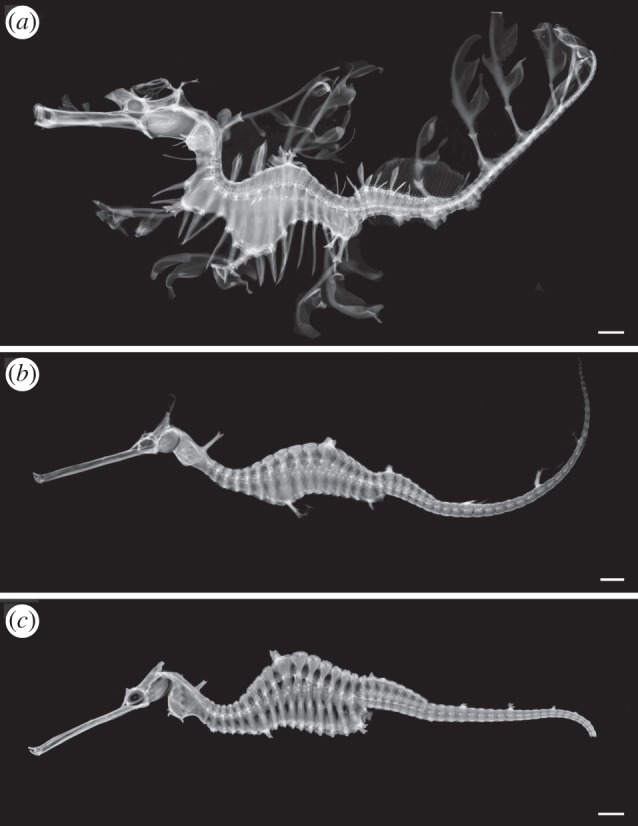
Comparison of the skeleton of the three species of seadragons. X-ray radiographs of (*a*) the leafy seadragon *Phycodurus eques*, SIO 04-28; (*b*) the common seadragon *Phyllopteryx taeniolatus*, SIO 84-300; (*c*) *Phyllopteryx dewysea* n. sp., holotype WAM P33223.002. Scale bars, 1 cm.
